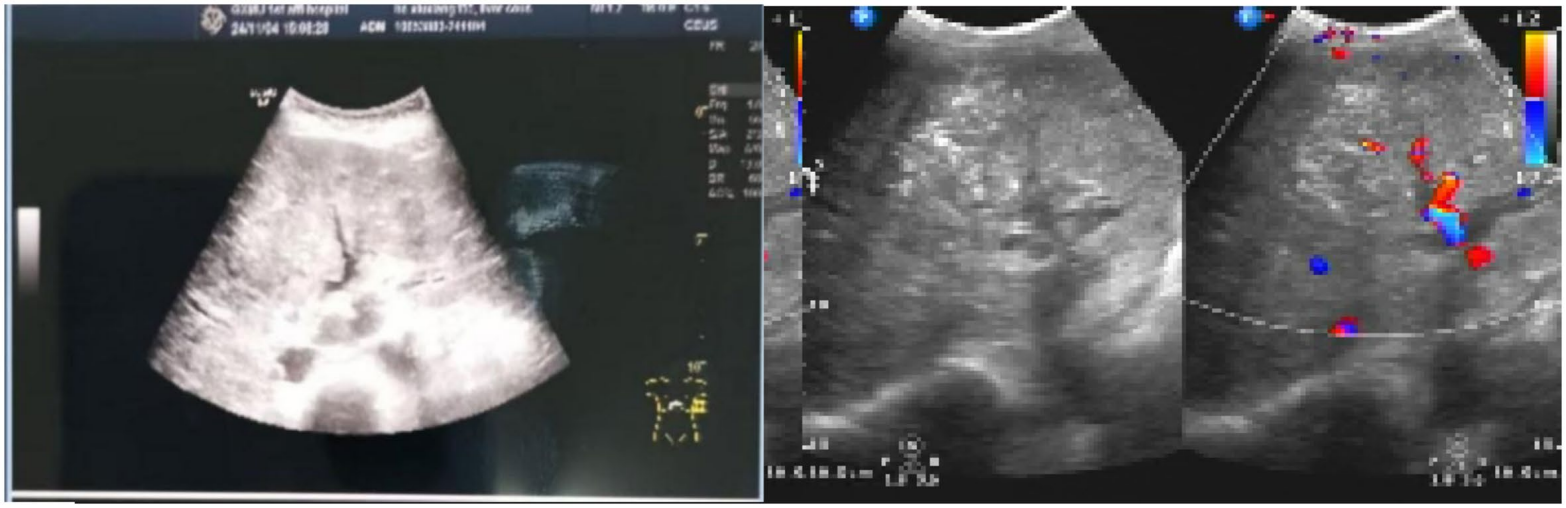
Abdominal ultrasonography before the initiation of the external application of Chinese medicine and moxibustion therapy, showing diffuse liver lesions, right branch embolism of the portal vein, and ascites.
